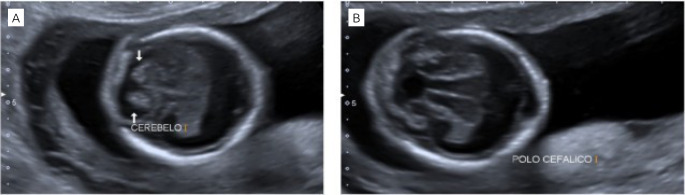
Obstetric ultrasound showing neurological alterations in Patau syndrome: (1A). Presence of a single ventricle and fusion of the thalamus; (1B). Cerebellar vermis agenesis.
